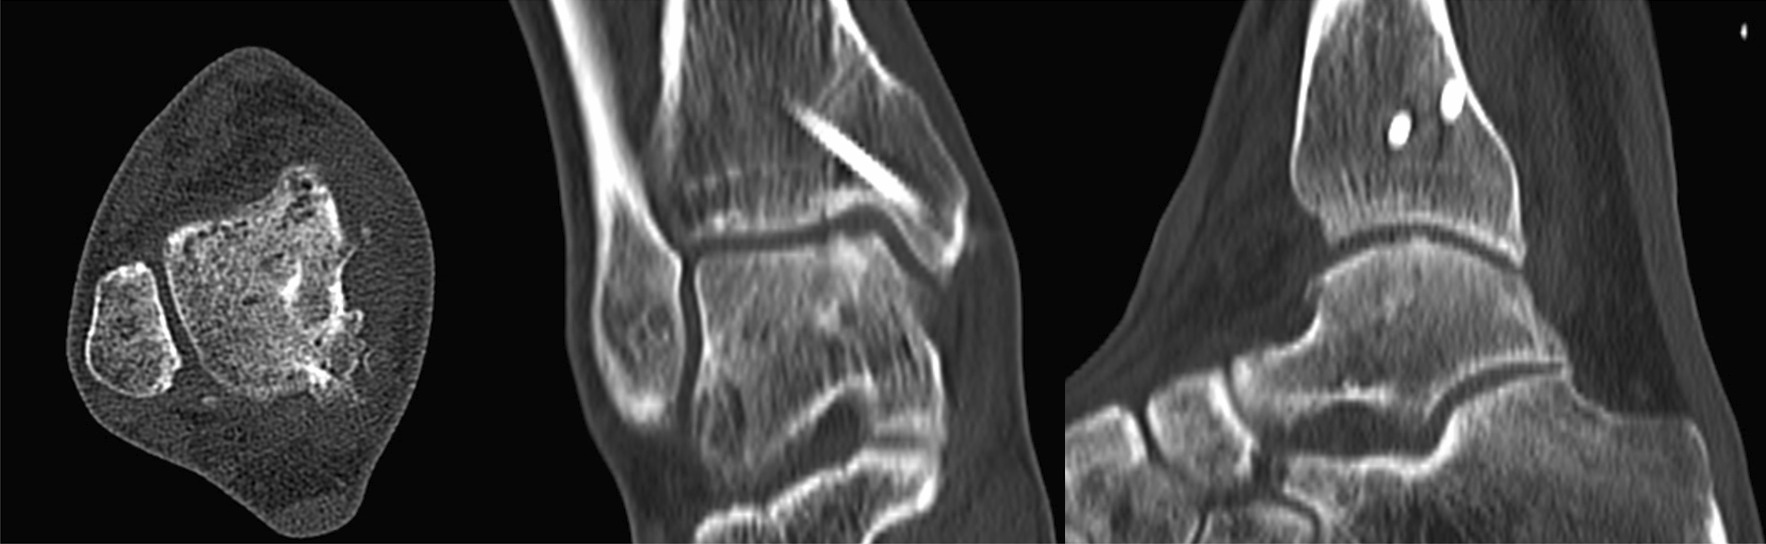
Postoperative CT shows medial malleolus osteotomy and talus bone graft have healed
